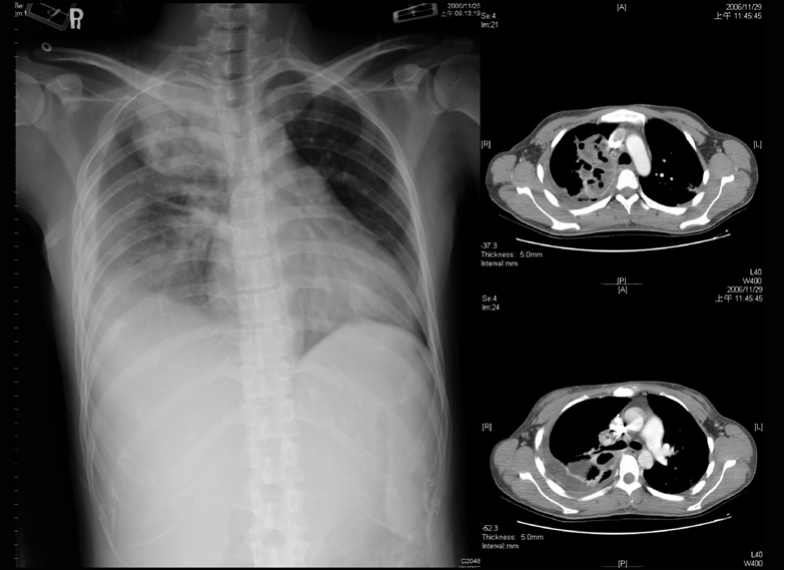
The chest radiograph and computed tomography showed pleural empyema with lung abscess.
